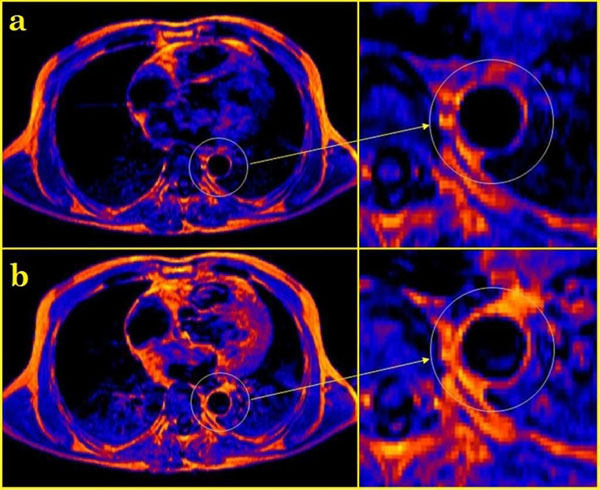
MRI in patient with extensive atherosclerosis and myocardial infarction of the left ventricular - a - T1-weighted image before contrast injection, b - T2-weighted image after contrasting - diffuse accumulation syndrome of contrast paramagnetic material in the aortic wall.
